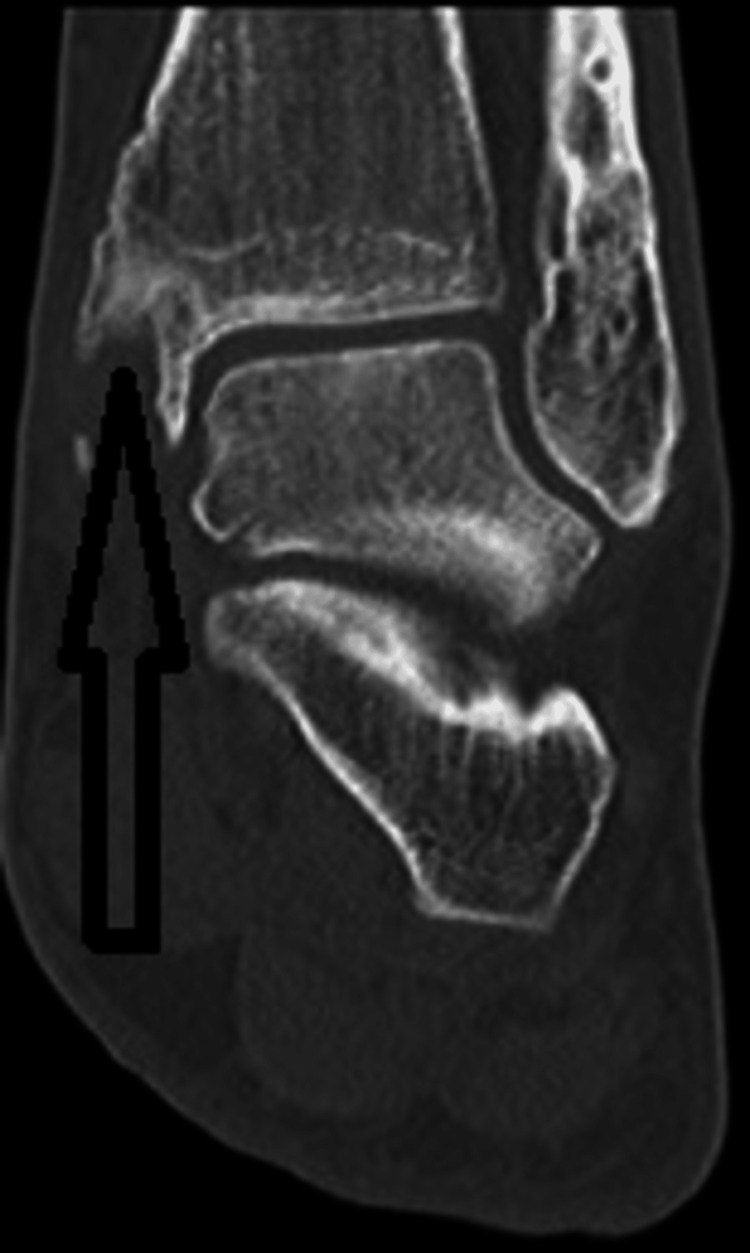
CT scan of the ankle showing the formation of an osseous tunnel in the medial malleolus
